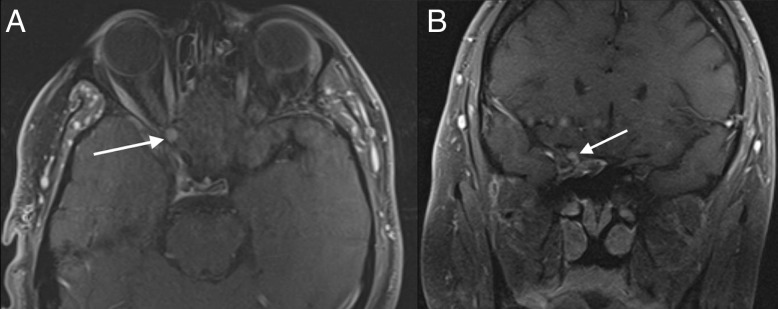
Postoperative T1-weighted MRI with contrast Axial view demonstrating a roughly stable enhancing schwannoma following decompression and biopsy as pointed out by the white arrow (A). Coronal view with the tumor again highlighted by the white arrow (B).
